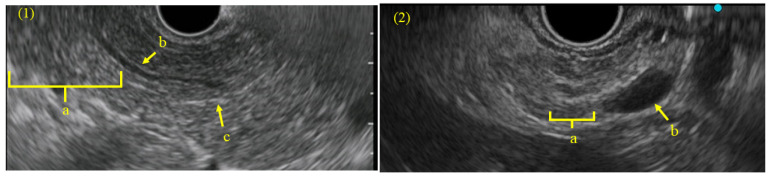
EUS findings according to the Rosemont Classification. (1) a. Hyperechoic foci without shadowing. b. Hyperechoic MPD margins. c. Stranding. (2) a. Lobularity without honeycombing. b. cyst. EUS: endoscopic ultrasonography, MPD: main pancreatic duct.
